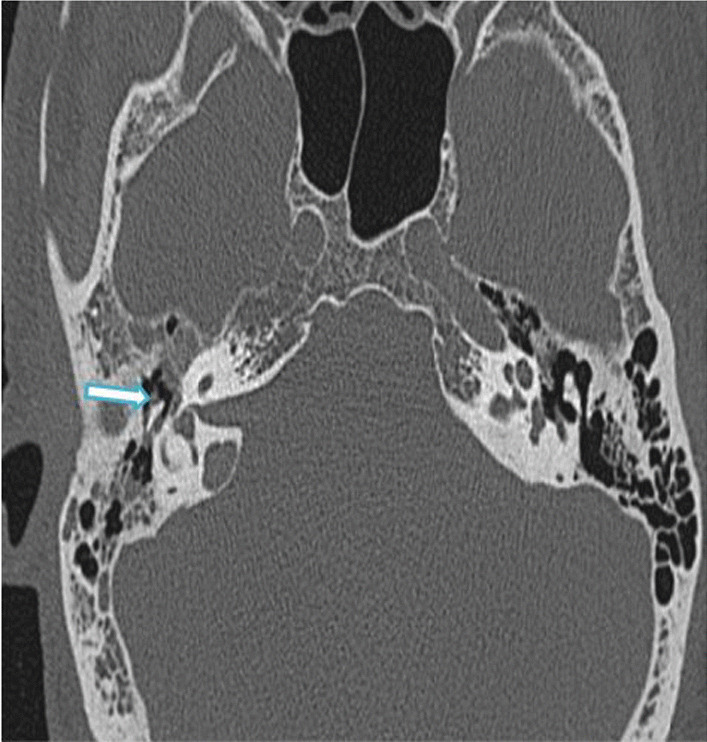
Axial HRCT temporal bone image of a 66-year-old male patient with complaints of otalgia and reduced hearing showing soft tissue attenuation in right middle ear and mastoid air cells with ossicular erosion of head of malleus (arrow)—invasive oto-mastoiditis
